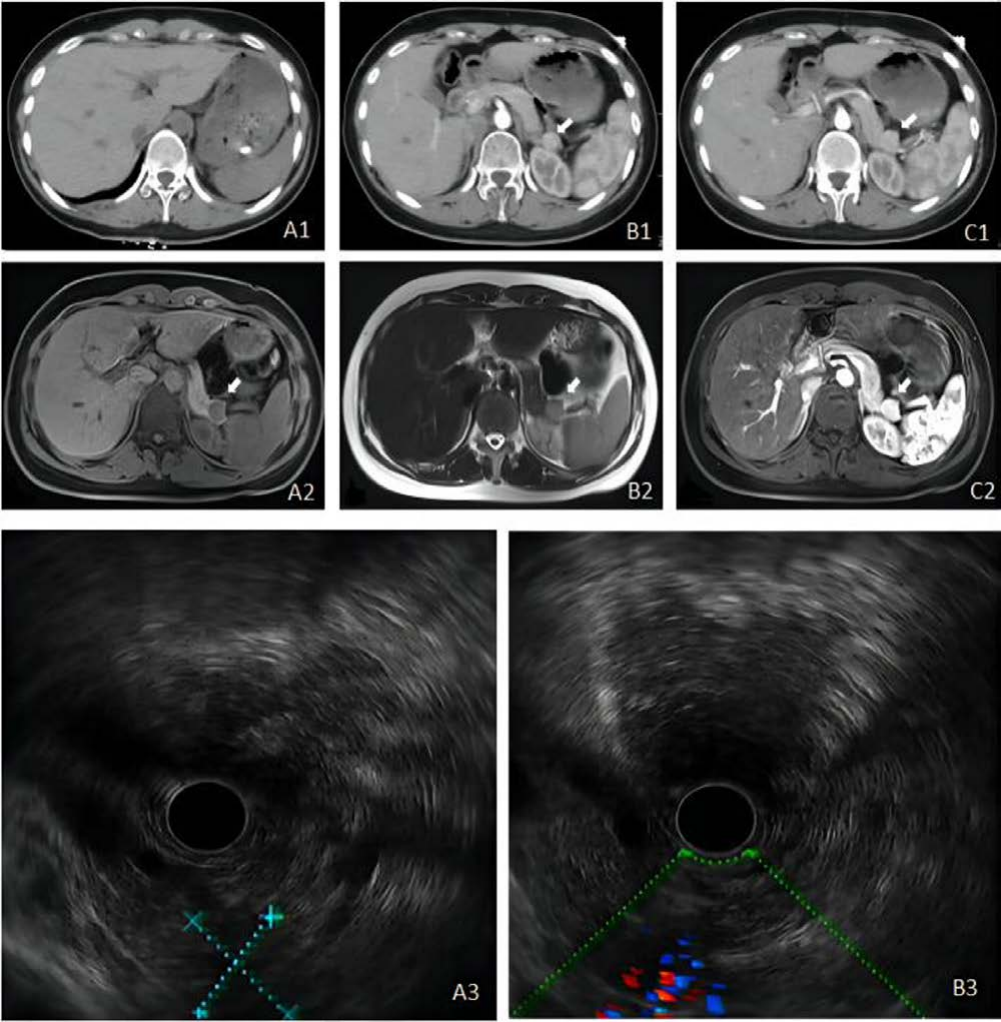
(A1, B1, and C1) Abdominal CT plain scan and enhanced scan of Case 1 indicating suspected lesion in the pancreas. (A2, B2, and C2) Pancreatic MRI of Case 1 showing blood supply of the pancreatic tail, indicating a neoplastic lesion and the high possibility of neuroendocrine tumors. (A3 and B3) Pancreatic abnormalities in endoscopy ultrasound examination of Case 1, including a pancreatic tail lesion indicating the possibility of a neuroendocrine tumor. CT = computerized tomography, MRI = magnetic resonance imaging.
